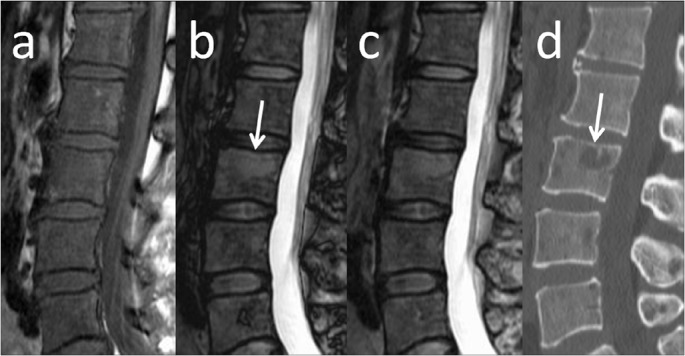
MRI of the lumbar spine in a false-negative case misclassified on the basis of β_2_-microglobulin. Sagittal MR images and sagittal reconstructed CT of a 52-year-old woman with symptomatic myeloma and a fat-signal fraction of 11% are shown. Serum creatinine level was 0.59 mg/dl, β_2_-microglobulin was 2.32 mg/l, and BMPC% from the iliac crest was 87%. The elevated β_2_-microglobulin might have resulted from renal impairment. a) T1-weighted imaging shows diffusely decreased signal intensity in bone marrow, suggesting replacement of fatty marrow by myeloma cells. A focal lytic lesion is also seen in the L3 vertebral body (arrows). b) Calculated out-phase image. c) Calculated in-phase image. d) Sagittal reconstructed CT.
